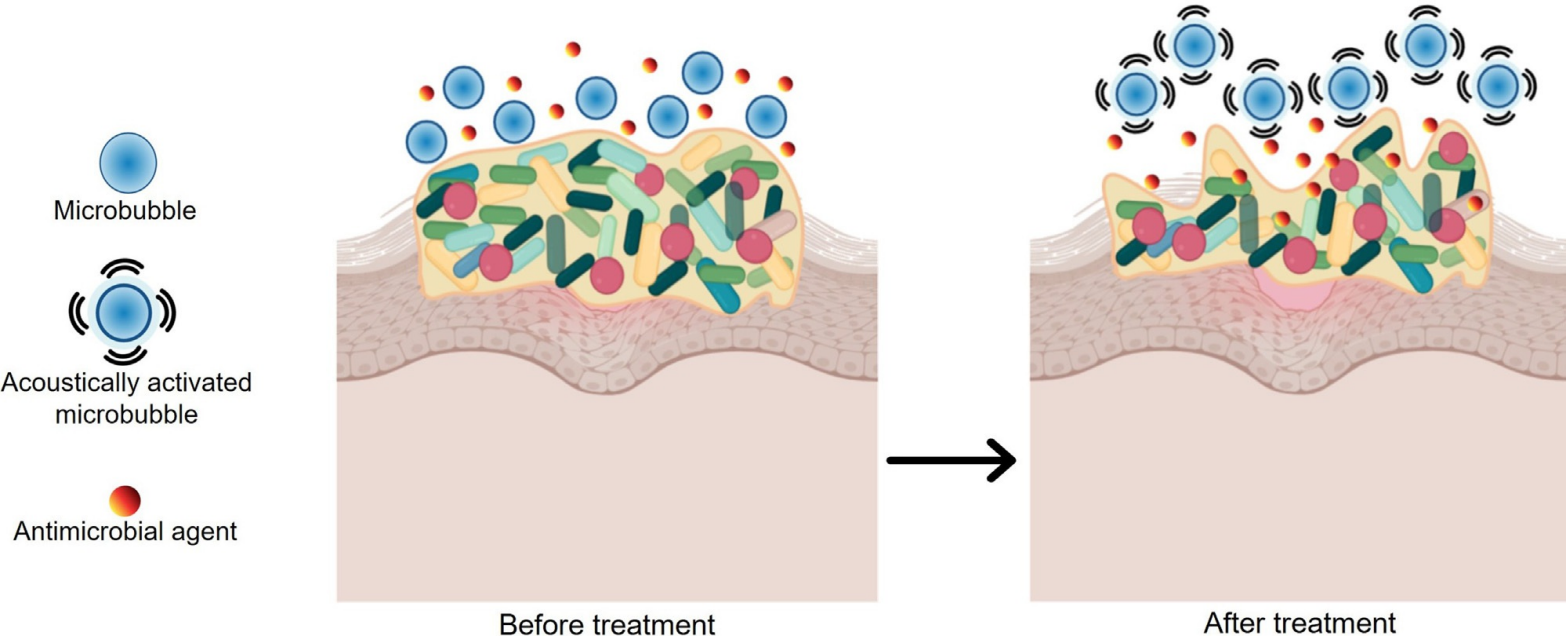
Concept of ultrasound‐mediated microbubble therapy. The microbubbles are acoustically activated after they are delivered and antimicrobials are applied (before treatment) to disrupt the biofilm and allow for the penetration of antibiotics (after treatment).
